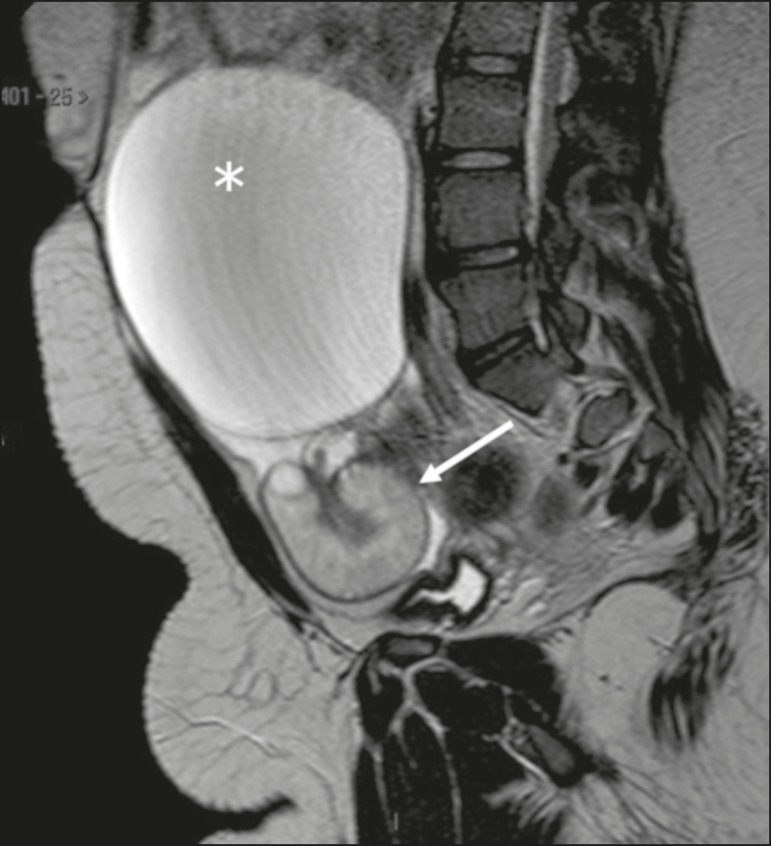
Sagittal T2-weighted MRI sequence. Thickened fallopian tube, measuring 1.4 cm in diameter (arrow). Note the large adnexal cystic formation (paraovarian cyst, asterisk).
